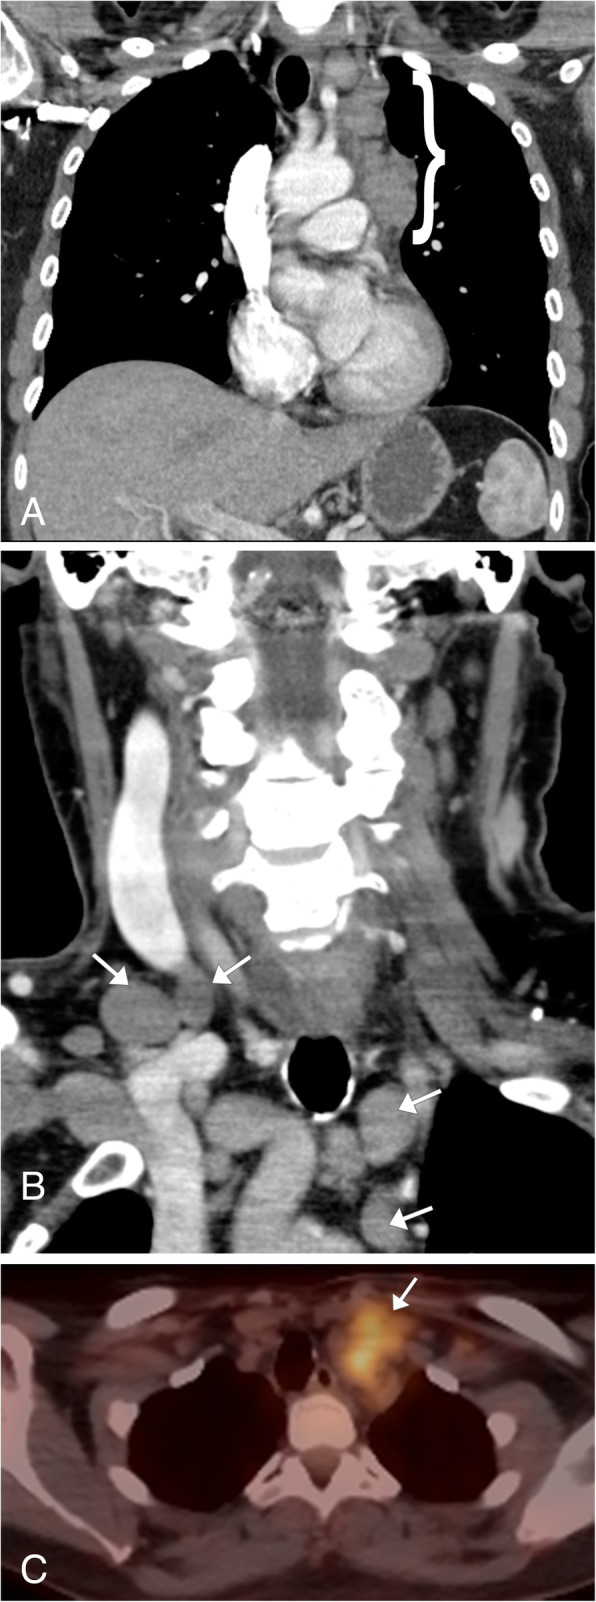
Lymphoma. a Coronal post contrast chest CT image with multiple enlarged left paratracheal and aortopulmonary window lymph nodes (bracket) in a patient with Hodgkin’s lymphoma. b Coronal CT post contrast image of the neck base shows multiple enlarged right neck base and left superior mediastinal lymph nodes in a patient with non-Hodgkin’s lymphoma (arrows). c Axial FDG-PET/CT image at the level of the thoracic inlet in the patient with Hodgkin’s lymphoma demonstrates hypermetabolic activity of the enlarged left paratracheal lymph nodes (arrow)
